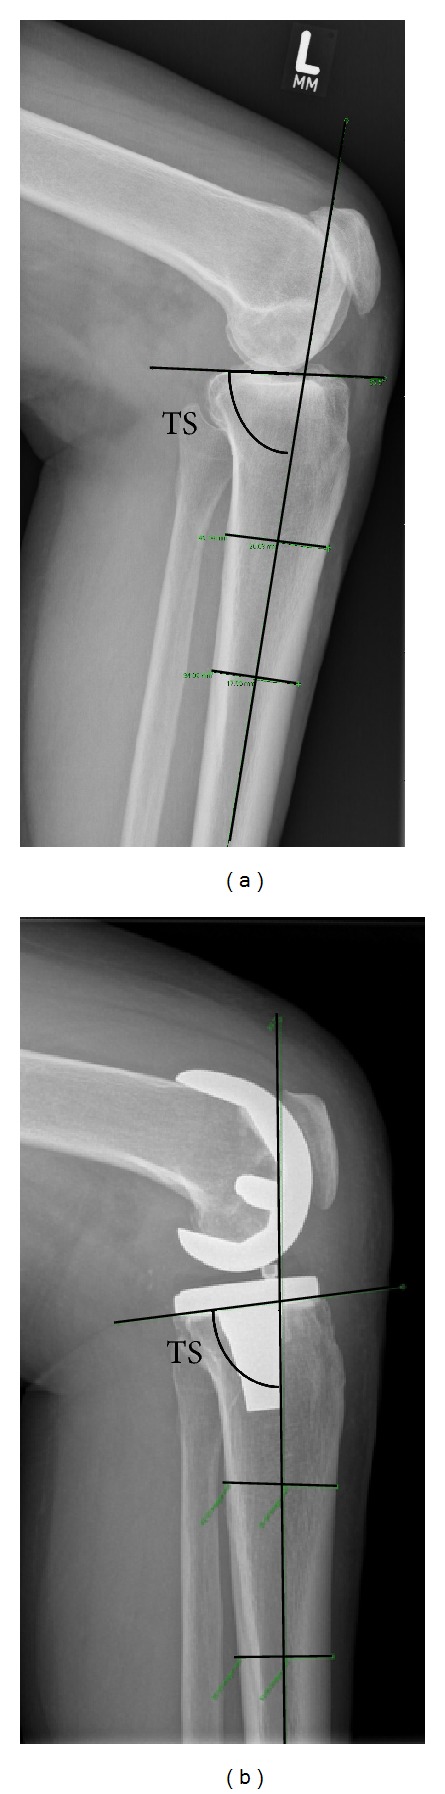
Tibial slope was measured in lateral radiographs of the knee joint preoperatively (a) and postoperatively (b) and was defined as the angle between the proximal tibial anatomical axis and the tibial plateau in the sagittal plane as proposed by Dejour and Brazier [16, 17].
